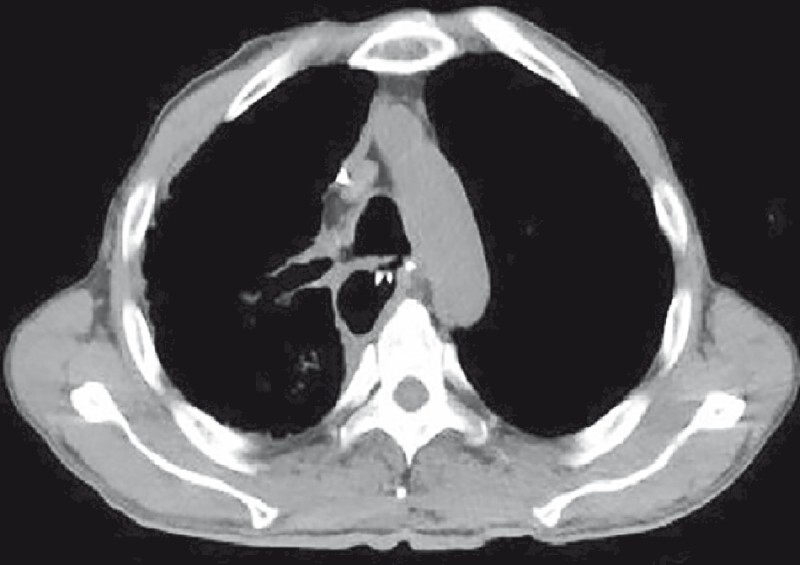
Chest computed tomography indicated that the bronchus exhibits communication with both the thorax and stomach.
